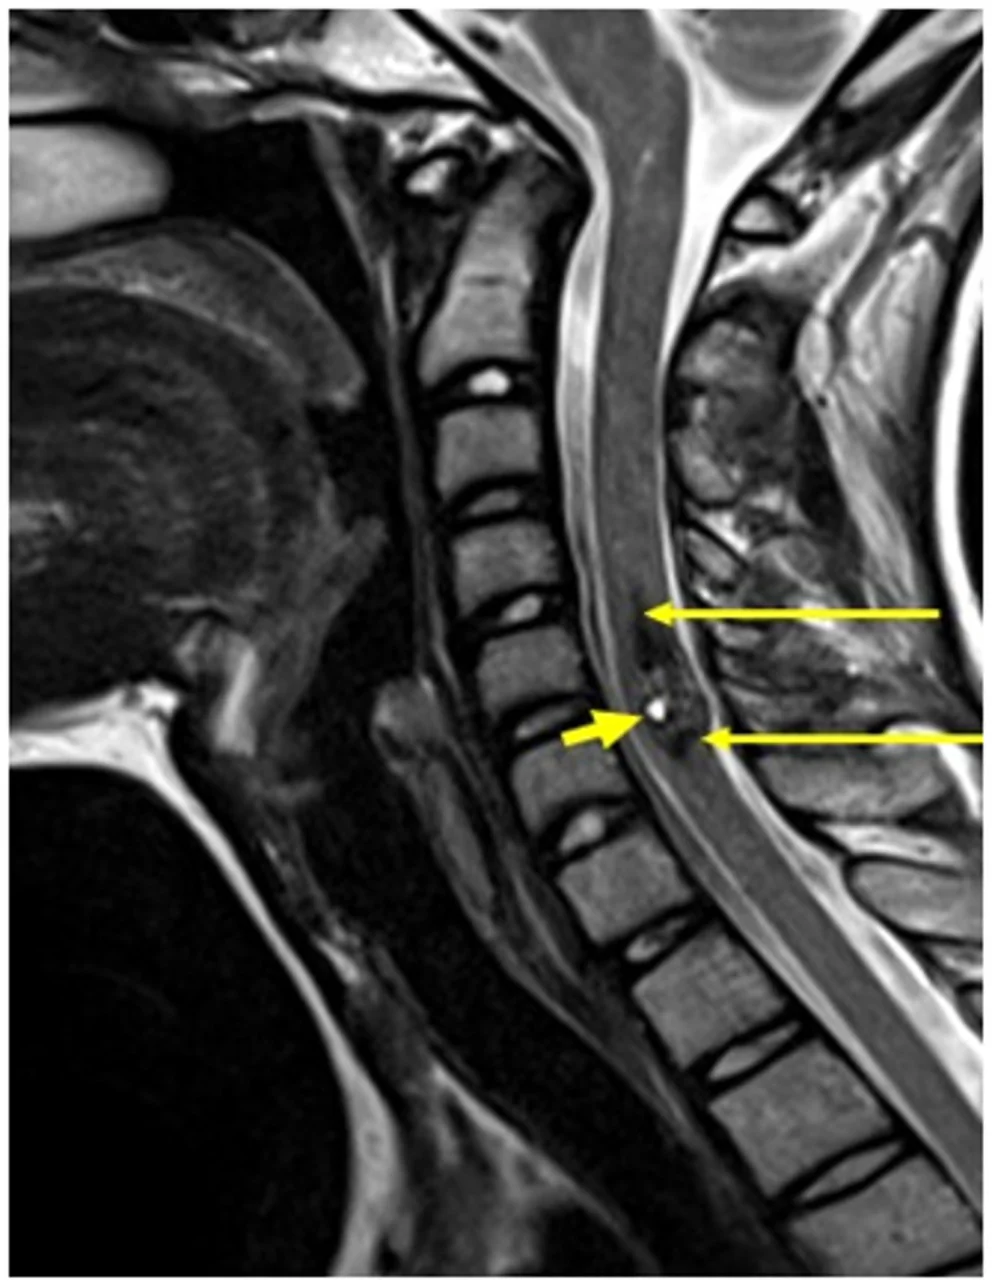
Sagittal T2‐weighted MRI of the cervical spine demonstrating an intramedullary lesion with a central hyperintense core (arrowhead) surrounded by a hypointense hemosiderin rim (arrows), consistent with a spinal cord cavernous malformation.
